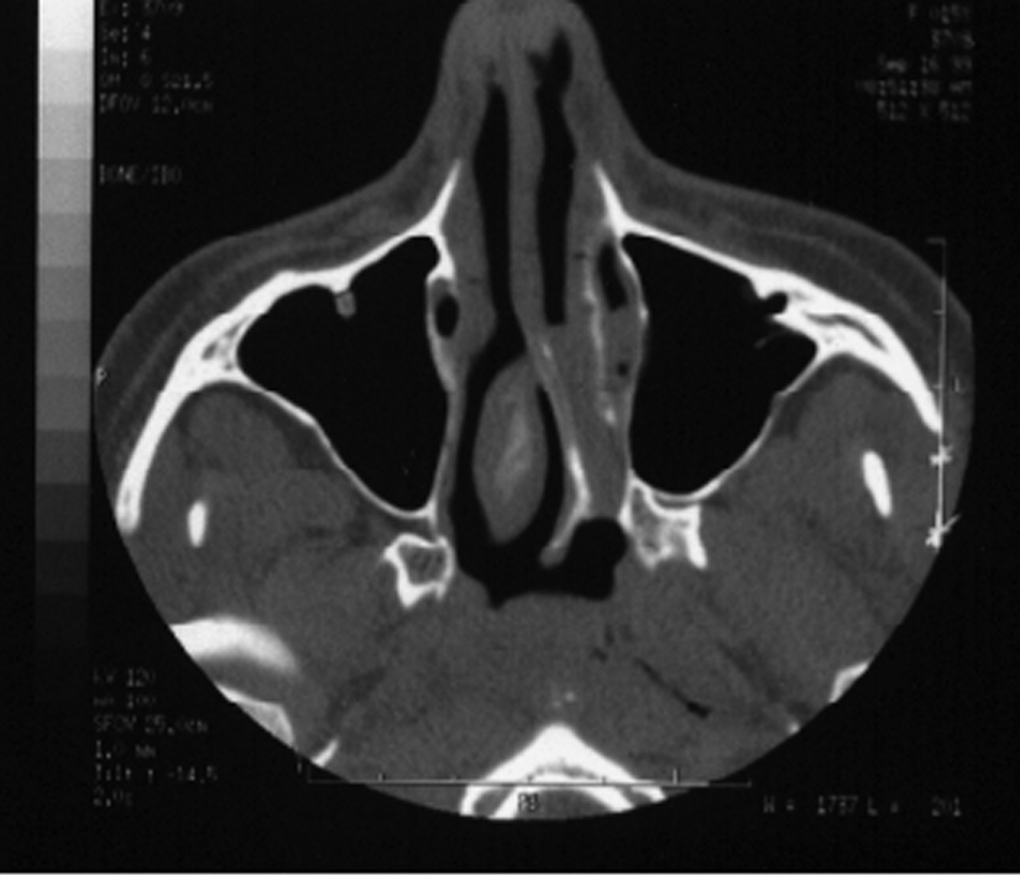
A case with CT scan characteristics similar to the one shown in Photo 1, left side choanal atresia, symmetrical maxillary sinuses and no sinus disease.
